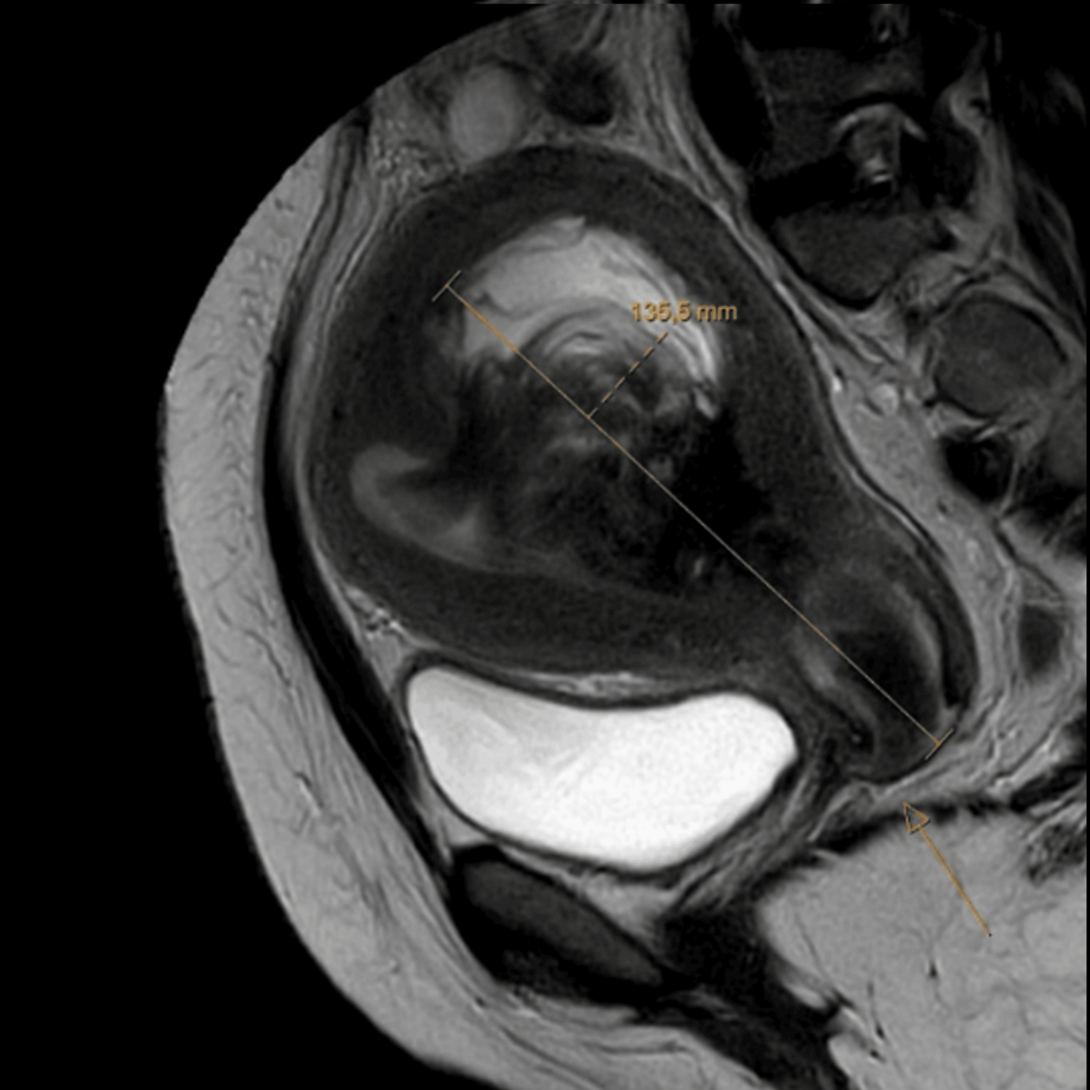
MRI of myoma nascent during hospitalization The arrow indicates the expelled portion of the nascent pyomyoma in the vagina. Known large heterogeneous T2-intermediate intense mass endocavitary within the uterine cavity, which is currently completely prolapsed into the endocervical canal and already protruding into the proximal vagina: differential diagnosis includes pedunculated endocavitary myoma versus endometrial polyp. The lesion is completely hypovascular. Spontaneous T1-hyperintense rim surrounding the lesion, compatible with endocavitary blood. Reactive enlarged lymph node in the left iliac region. Otherwise, no enlarged lymph nodes. No evidence of abscess formation in the abdomen.
